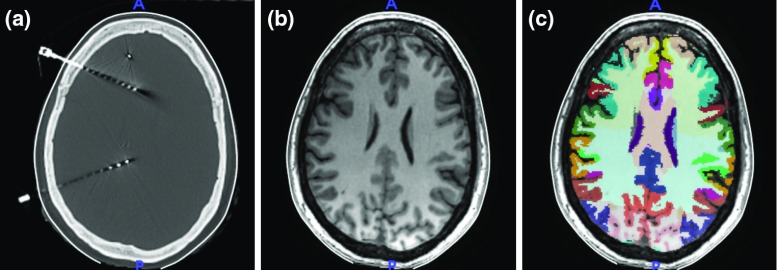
Input images: a post-SEEG implantation CT, b MRI T1, and c parcellation
